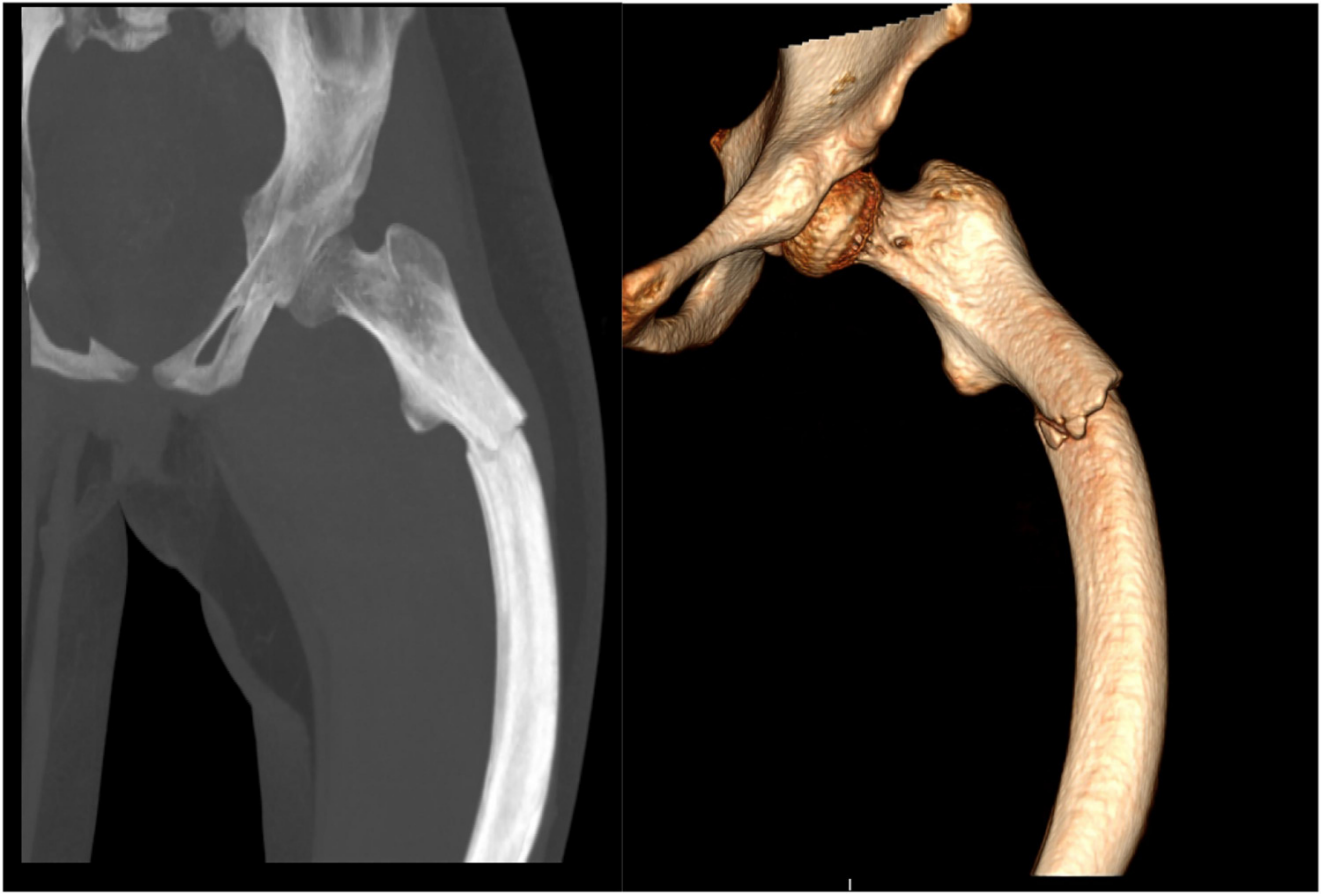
Comprehensive CT 3D reconstruction showing a left proximal femoral fracture, accompanied by severe left femoral deformity.
